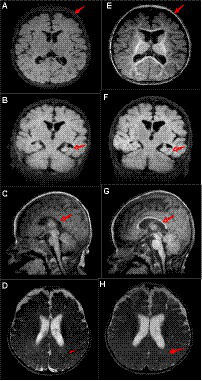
Patient’s brain MRI. At 4 months of age, (A) the T1 axial view shows brain atrophy; (B) the T1 coronal view shows reduced left hippocampal volume; (C) the T1 sagittal view shows a slightly thin corpus callosum; and (D) the T2 axial view shows impaired myelin development. At 14 months of age, (E) the T1 axial view shows widened extracerebral spaces; (F) the T1 coronal view shows reduced left hippocampal volume; (G) the T1 sagittal view shows a slightly thin corpus callosum (arrows, similar to C); and (H) the T2 axial view shows impaired myelin development (arrows, similar to D). Compared to 4 months of age, at 14 months, there is deepening of the cerebral sulci, increase relative volume of the lateral ventricles, and a reduction in the left hippocampal volume similar to before.
